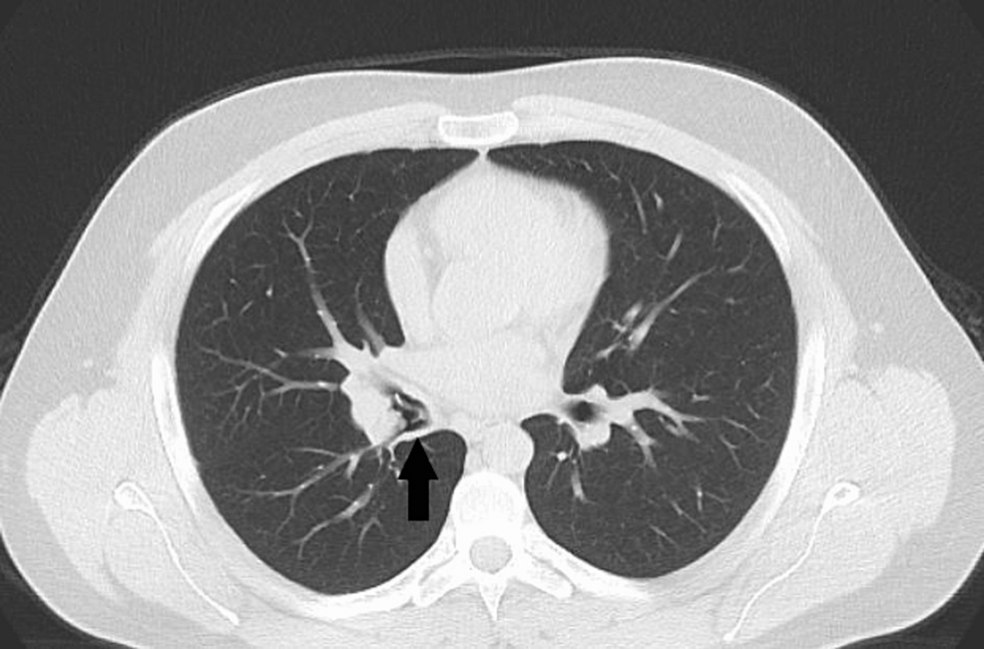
CT of the chest The arrow shows the foreign body in the right bronchus intermedius.
